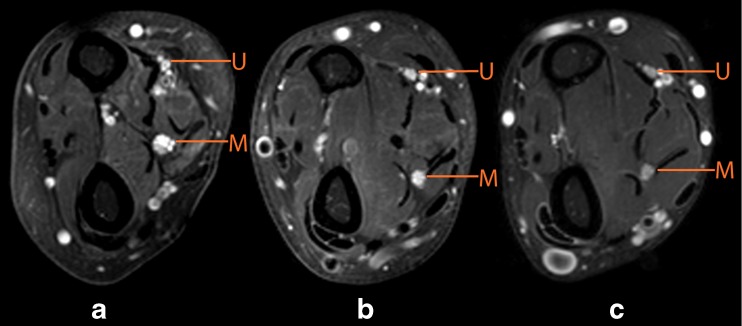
Axial plane of T2-weighted scans of the forearm with the median (M) and ulnar (U) nerves. a Multifocal motor neuropathy (MMN) patient with enlargement of the median nerve, (b) amyotrophic lateral sclerosis (ALS) patient and (c) healthy control
